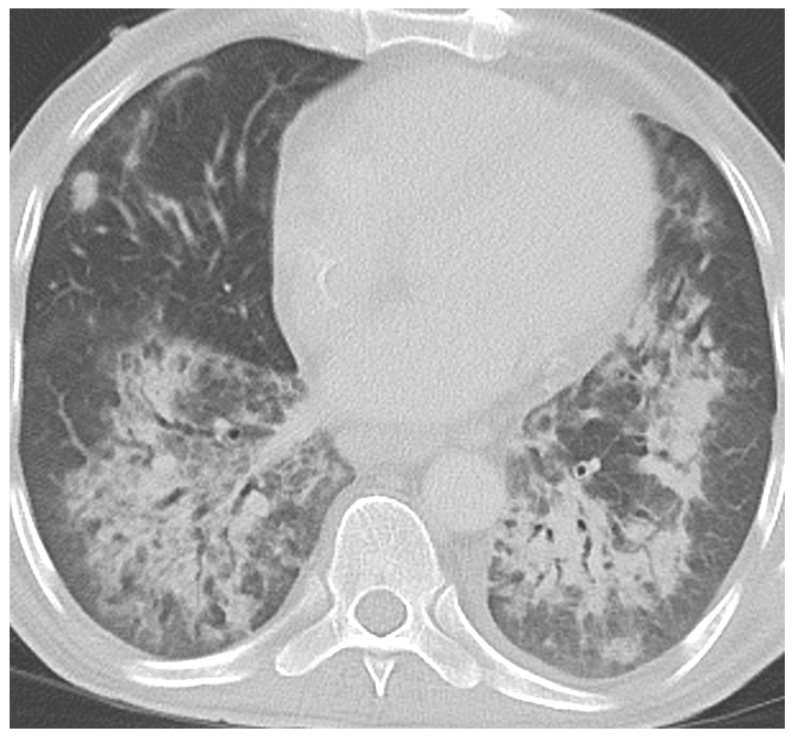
Chest CT: bilateral perihilar infiltrates and ground-glass opacities with subpleural sparing, without specific lobar predilection, in a patient with AIDS, consistent with *Pneumocystis jirovecii* pneumonia (PJP). Representative image from the anonymized institutional radiology archive of University Hospital Frankfurt.
